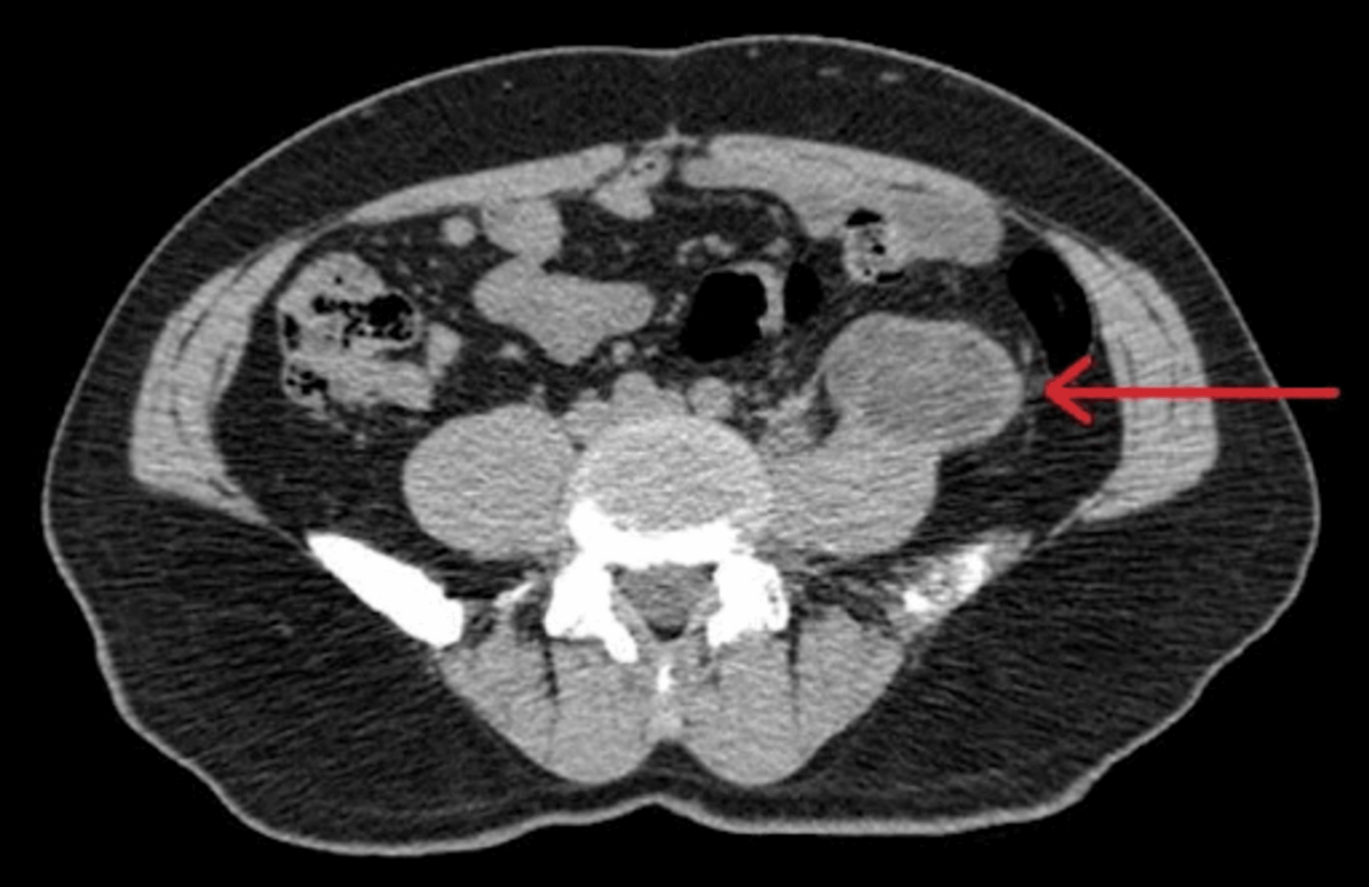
CT scan without contrast. Axial view (red arrow) showing multiseptated left psoas cyst.
